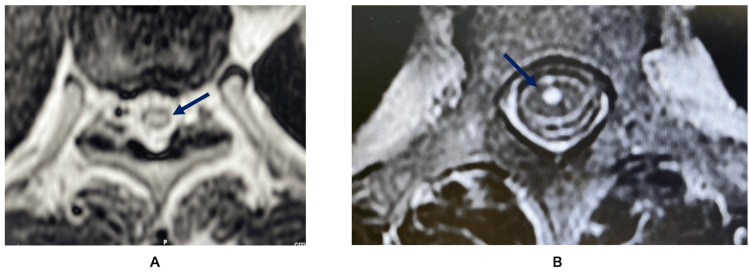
MRI image of edema. (A) Arrows indicate a bilateral edema in case 1; (B) arrows indicate an edema of the anterior horn of the spinal cord in case 9. MRI, magnetic resonance imaging
